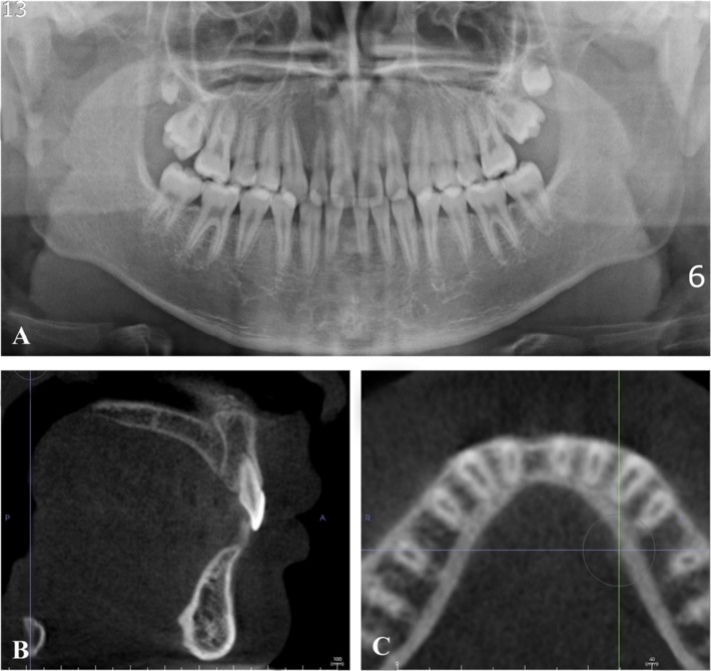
Pretreatment radiographs. A. Pretreatment Panoramic radiograph. B and C. Pretreatment sagittal and axial CBCT images. Because buccal plate of alveolar bone was concave, buccal placement of C-implant was inevitable.
